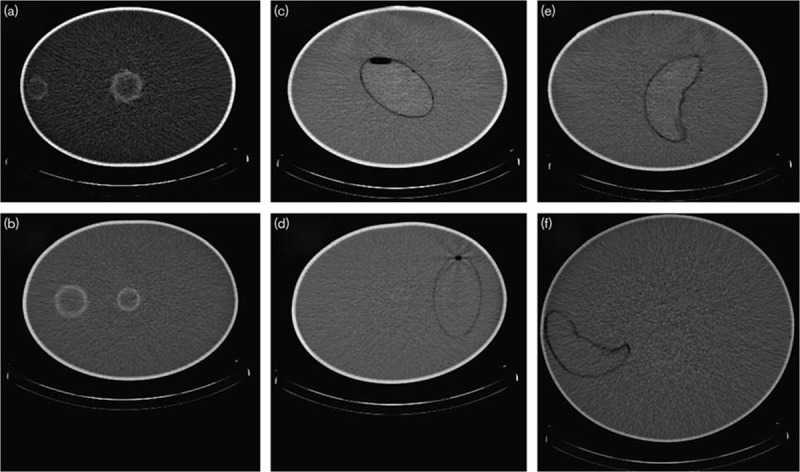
Computed tomography (CT) scans showing the positions of the inserts. (a) 113 ml sphere central, 16 ml sphere outer; (b) 16 ml sphere central, 113 ml sphere outer; (c) Cristy and Eckerman (C&E) spleen central; (d) C&E spleen outer; (e) patient spleen central; (f) patient spleen anatomical.
